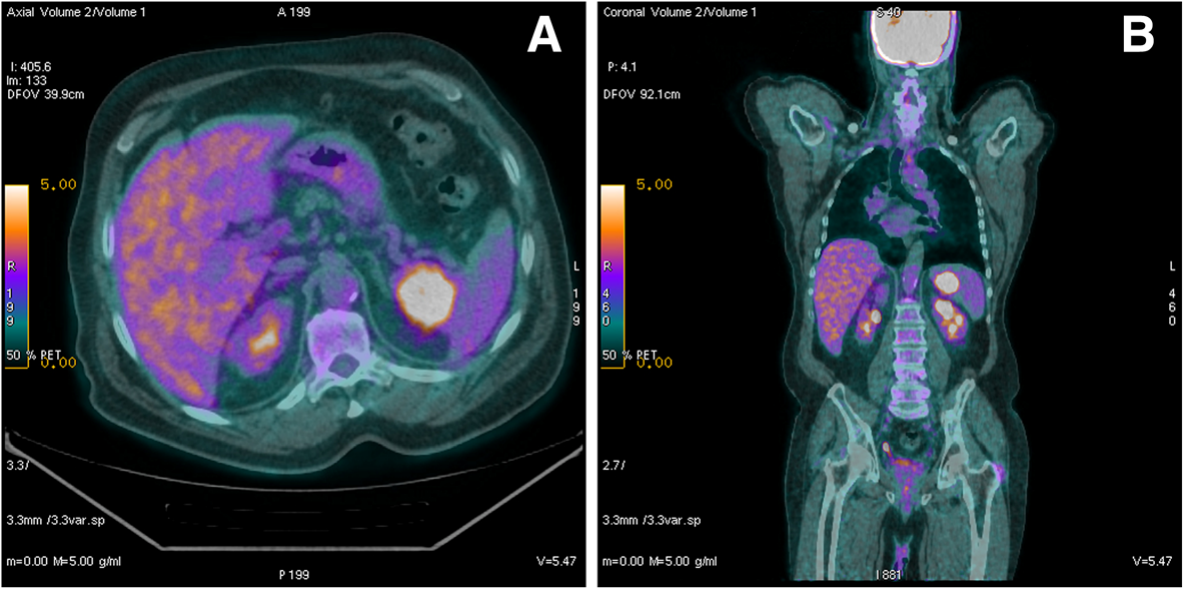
^18^F-FDG PET images of splenic metastasis. Axial section (A) and coronal section (B).
